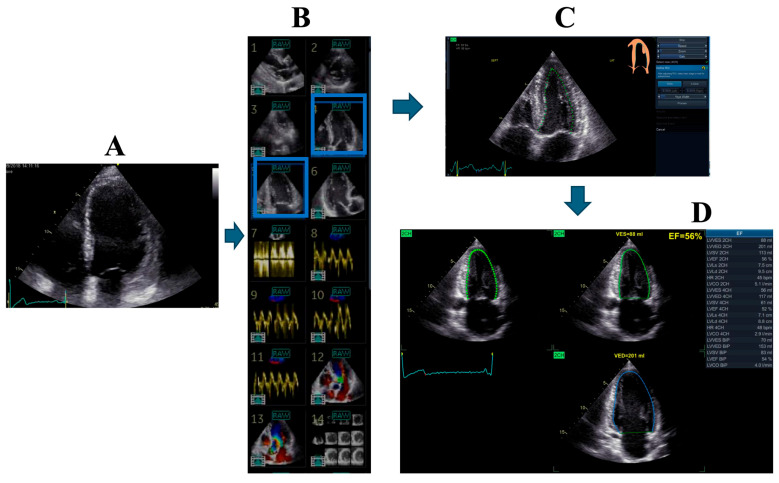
Example of AI-assisted echocardiographic analysis. AI-based algorithms are applied for automated segmentation and quantification of cardiac chambers from routinely acquired echocardiographic images. In this example, AI support is used to facilitate measurements and reduce operator dependency, rather than to enhance image quality at the acquisition stage. (A) Manual selection of a four- (or two-) chamber view image by the operator. (B) Automated retrieval by the software of the optimal corresponding cardiac cycle and frame rate from the stored study images (highlighted in blue). Panels 1–6 show 2D echocardiographic views; 7–11 spectral and tissue Doppler; 12–13 color Doppler; and 14 serial short-axis views derived from the apical long-axis. (C) Automatic endocardial border delineation in both diastole and systole for the selected views. (D) Automated calculation of LV volumes and function, including ejection fraction, stroke volume, and cardiac output.
